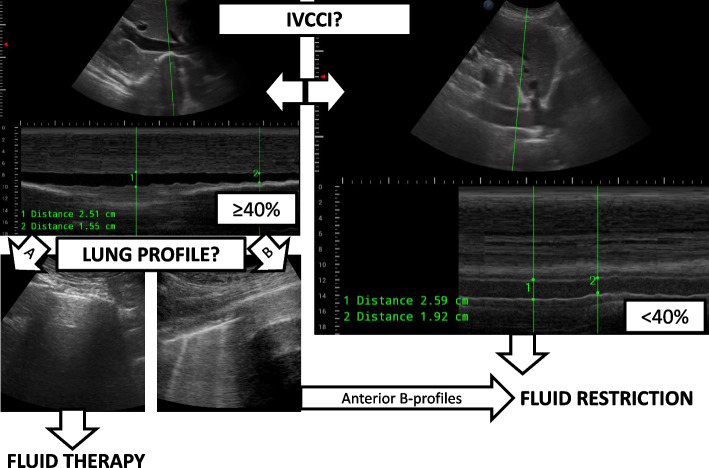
The decision tree and the typical findings of the preoperative ultrasounds
